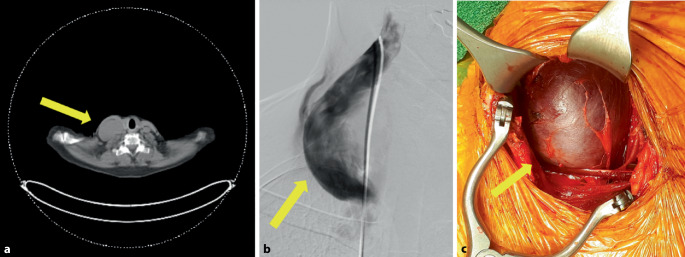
Fall 3: Venöses Aneurysma der V. jugularis interna rechts. a Darstellung des Aneurysmas mittels F‑18-Cholin-PET/CT (*gelber Pfeil*), b Selektive phlebographische Darstellung des V.-jugularis-Aneurysmas (*gelber Pfeil*), c Intraoperativer Befund des Aneurysmas (*gelber Pfeil*)
